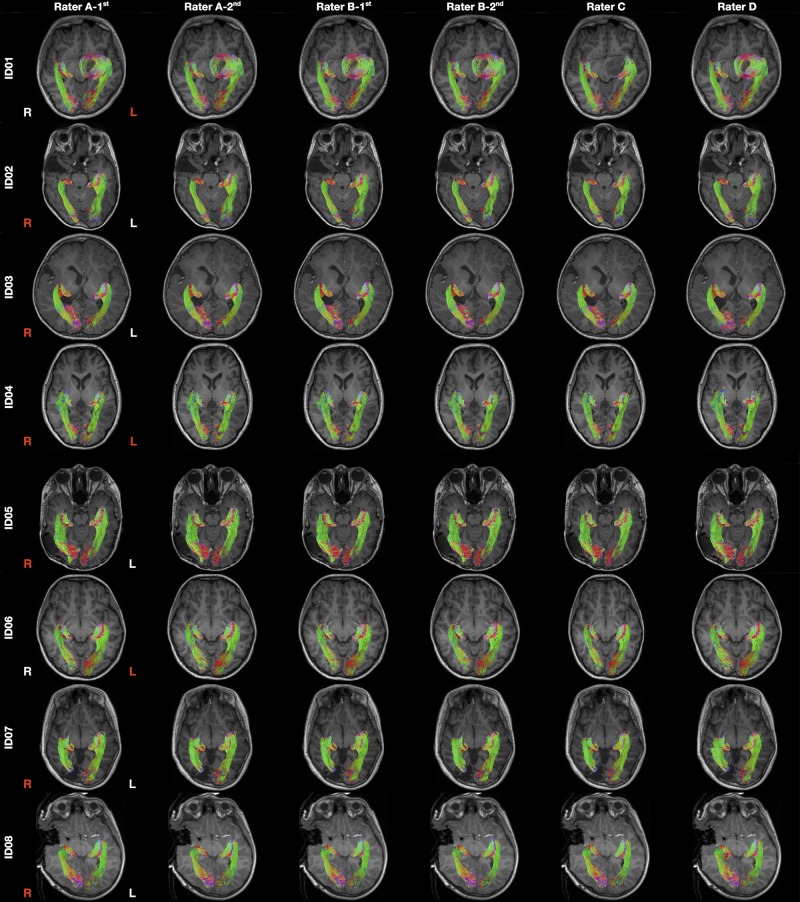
Final optic radiation tractography images generated from all raters. The tractography images are color coded by tract directions: left-right (red), superior-inferior (blue), and anterior-posterior (green). The MRI images are displayed in radiology convention. L, left; R, right. Rater A (B)- first/second = first/second tractography attempt by rater A (B). Hemisphere side colored by lesion/pathology: red, lesional; white, non-lesional.
